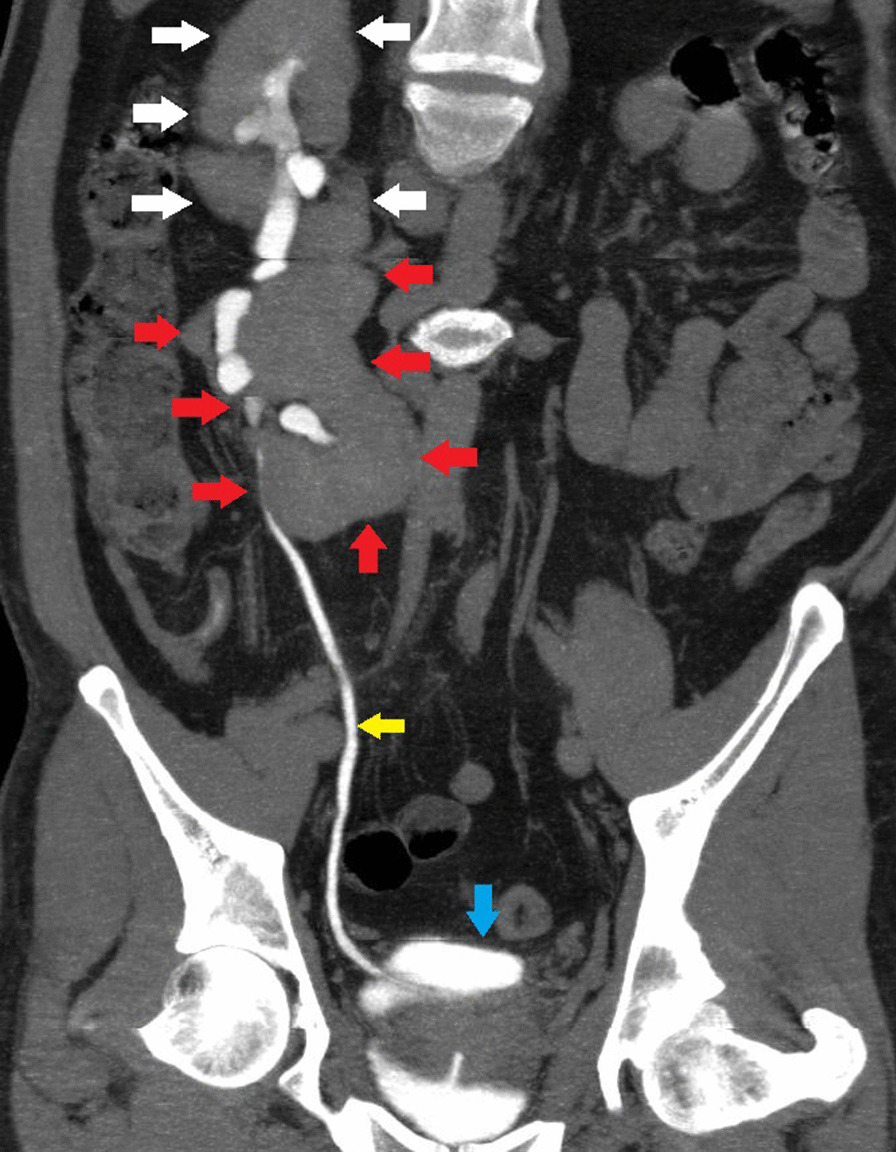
Contrast-enhanced computed tomography coronal section delayed phase curved reformatted images reveal two moieties in the right kidney (shown by white and red arrows), with only one ureter present (yellow arrow). The urinary bladder is shown by the blue arrow. The left renal fossa and other potential ectopic sites show the absence of the kidney
